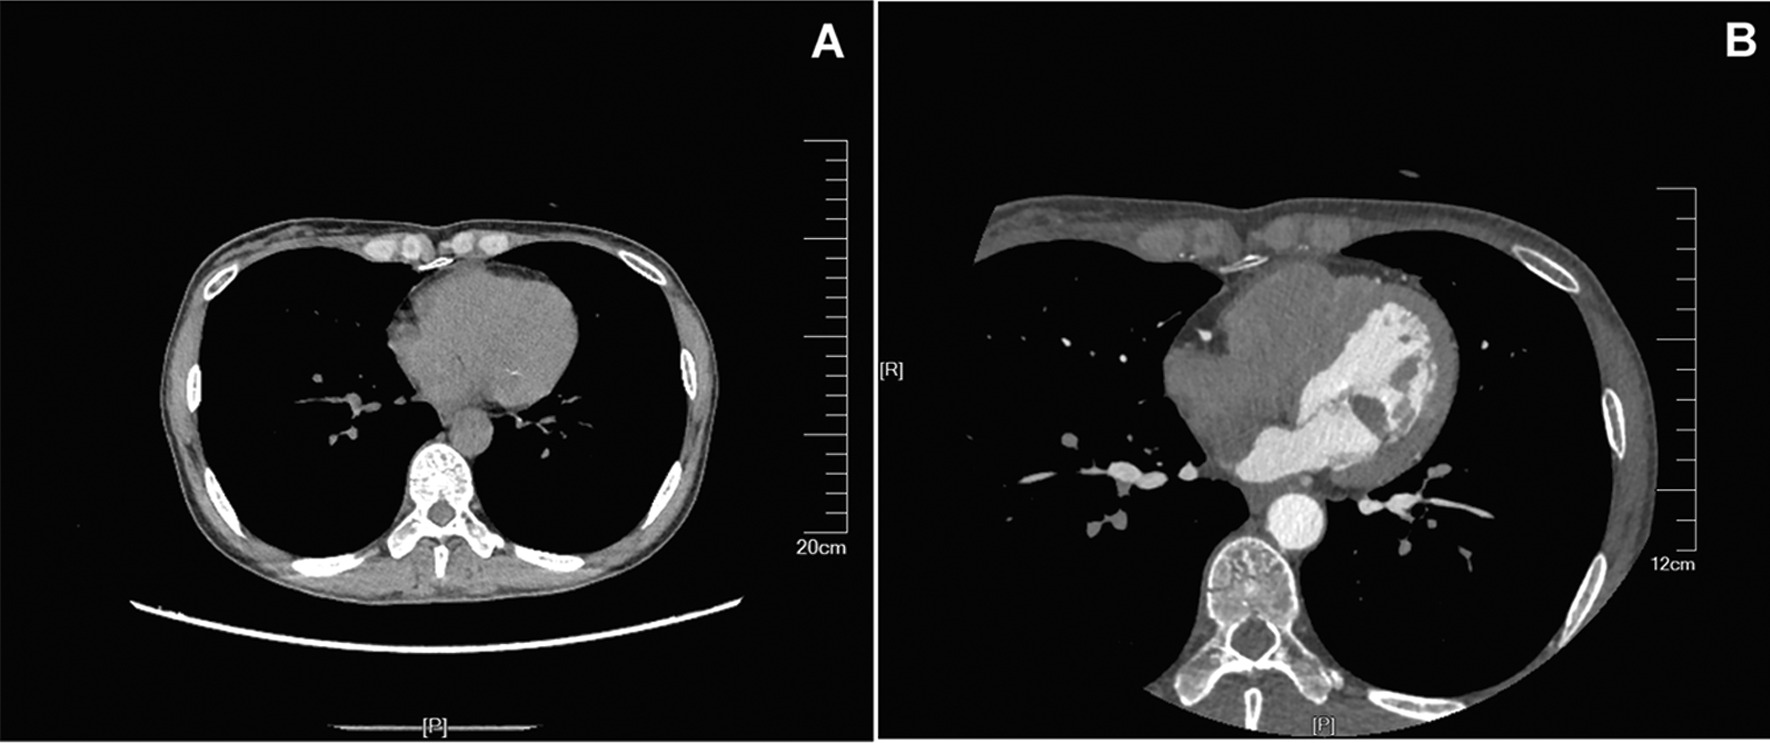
A Chest CT revealing a calcified mass lesion in the left ventricle. B Contrast-enhanced coronary CT angiograms revealing a homogeneous low density mass with punctate calcifications measured 19 mm × 8 mm and no significant enhancement
